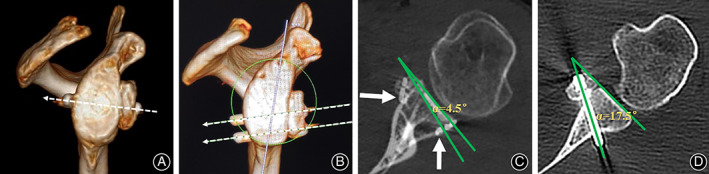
Mechanical stress direction of the coracoid graft: (A) Arthroscopic Latarjet technique with suture‐button fixation; (B) Arthroscopic Latarjet technique with screw fixation. Angulation of fixation devices relative to joint line in axial plane; (C) Arthroscopic Latarjet technique with suture‐button fixation (white arrows pointed to the two suture buttons based on picture overlap technique); (D) Arthroscopic Latarjet technique with screw fixation. The angulation was evaluated on the axial CT scan by measuring the angle (α) between the axis of the fixation device and the glenoid fossa.
